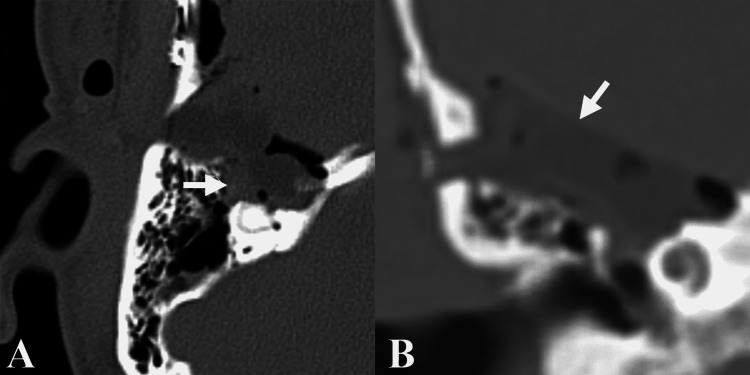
Postoperative imaging demonstrating resection. (A) Axial and (B) coronal computed tomography imaging obtained on postoperative day 1 demonstrating no residual perigeniculate mass; arrows point to fat graft used for reconstruction
